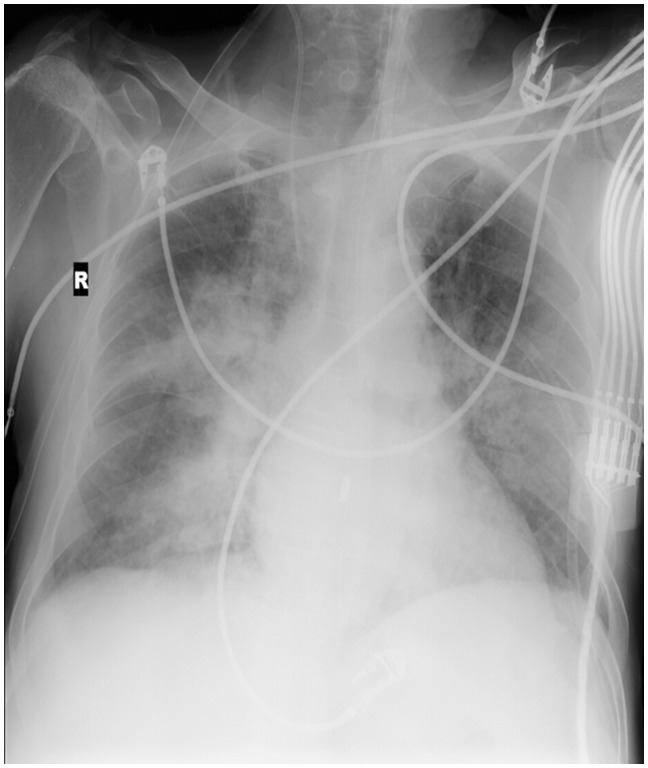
Acute respiratory distress syndrome. Chest radiograph in a patient with acute respiratory distress syndrome (admitted to intensive care unit) showing diffuse coalescent opacities and consolidation in both lungs.
